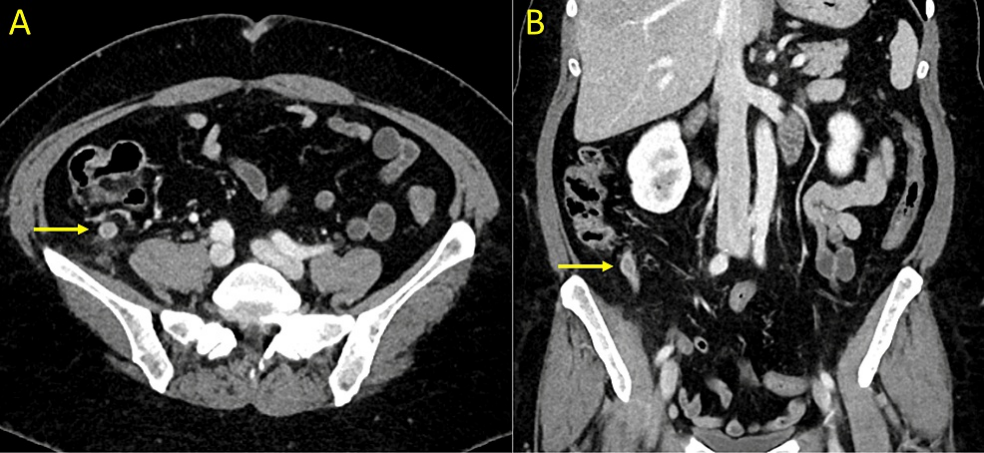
Three-week follow-up computed tomography (CT) of the abdomen CT scan of the abdomen at the patient's three-week follow-up visit revealed a 2 cm walled-off collection abutting the tip of the appendix (yellow arrows). (A) Axial view. (B) Coronal view.
